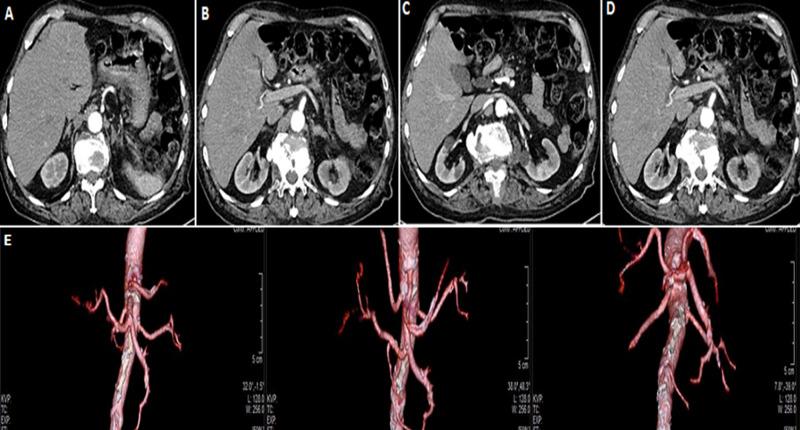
A) angio-CT abdominal Scan demonstrated absence of complete celiac trunk bipode; B) common hepatic artery (CHA); C) left hepatic artery (LHA); D) right hepatic artery (RHA) arising from superior mesenteric artery (SMA); E) 3D image: superior mesenteric artery (SMA) + common hepatic artery (CHA), celiak bipode
